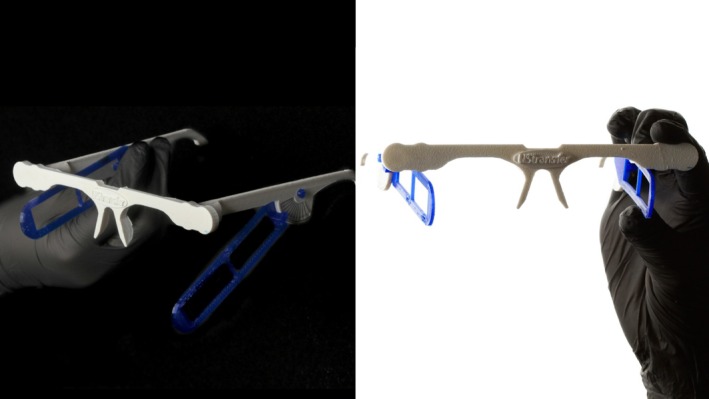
Positioning device used for standardized ultrasound acquisitions. The custom‐designed glasses‐like structure features flexible arms that ensure consistent probe placement across different time points, minimizing variability in dermal thickness measurements.
